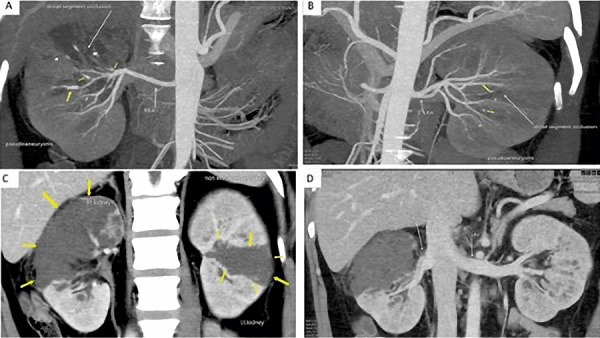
Contrast CT abdomen showing bilateral small pseudoaneurysms in the segmental branches of the renal arteries (A, B) with bilateral renal infarcts (C) with patent bilateral renal vein (D).
